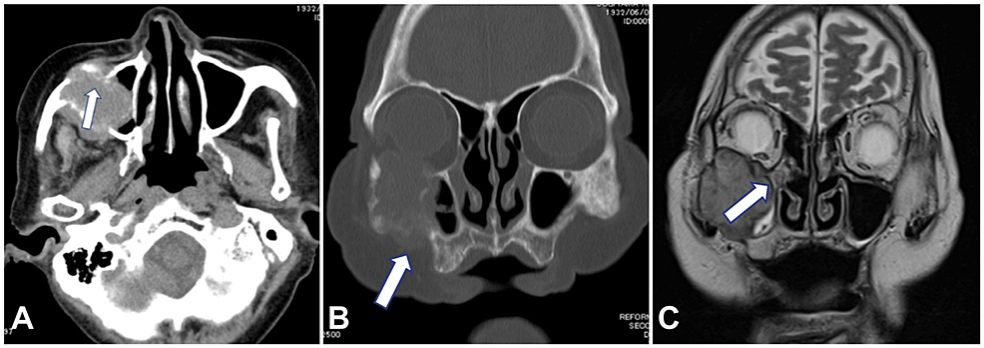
Imaging findings of the paranasal sinuses A) Horizontal section sinus CT. The mass in the right maxillary sinus is infiltrating into the maxillary sinus (arrow). B) Coronal section sinus CT. The mass shadow in the right maxillary sinus is infiltrating outside the maxillary sinus bone (arrow). C) Coronal MRI T2-weighted image. The low-signal mass shadow extends into the right orbit. There is a slightly high-signal shadow in the right middle nasal canal that appears to be secondary inflammatory tissue (arrow). CT, computed tomography; MRI, magnetic resonance imaging.
